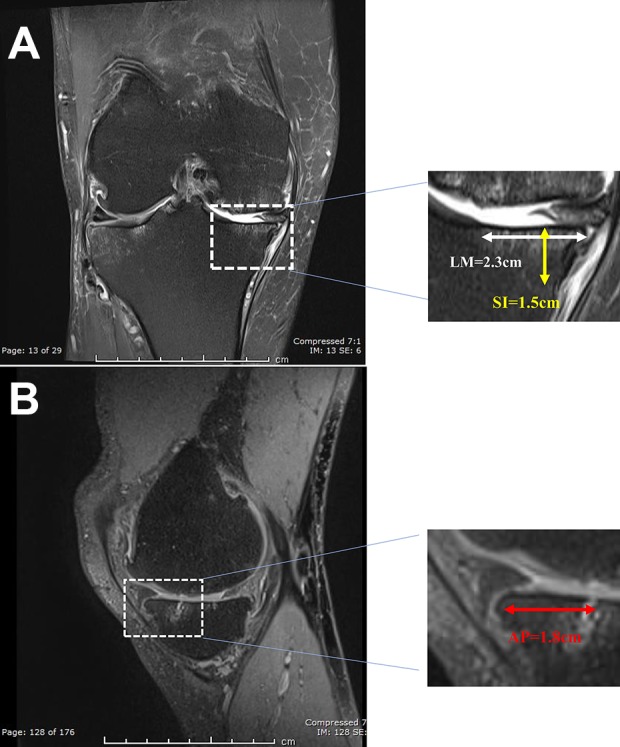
Measurement of the bone marrow lesion volume on MRI within the medial tibial plateau of patient A. (A) In the coronal image, the LM dimension (2.3 cm) and SI dimension (1.5 cm) of the lesion are measured. (B) In the sagittal image the AP dimension of the same lesion (1.8 cm) is measured. AP: anterior-posterior; LM: lateral-medial; MRI: magnetic resonance imaging; SI: superior-inferior.
